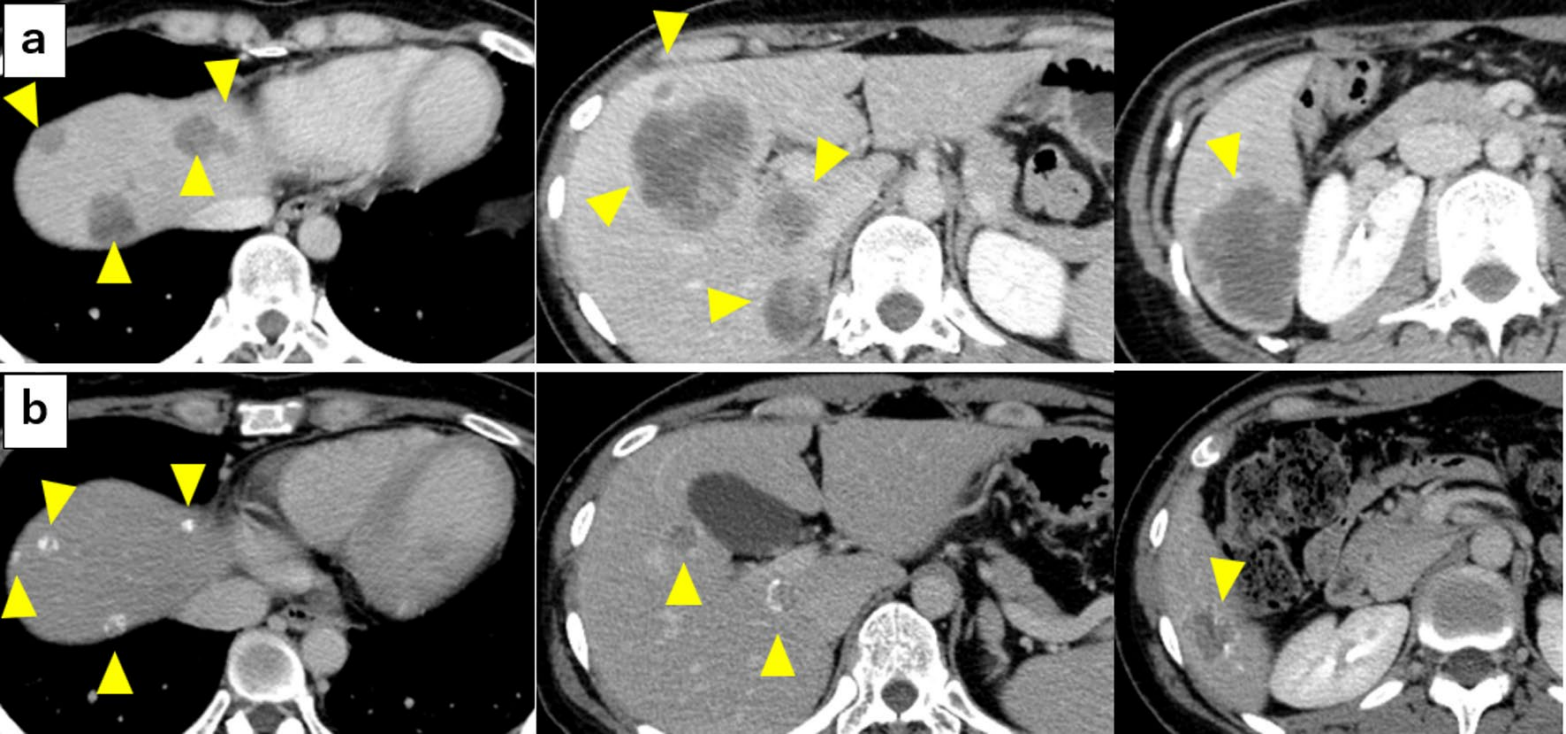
Abdominal CT findings before and after chemotherapy (15 cycles of FOLFOXIRI + bevacizumab). Yellow arrowheads indicate multiple liver metastases (a). After chemoradiotherapy, the liver metastases were significantly reduced in size and were calcified (b)
